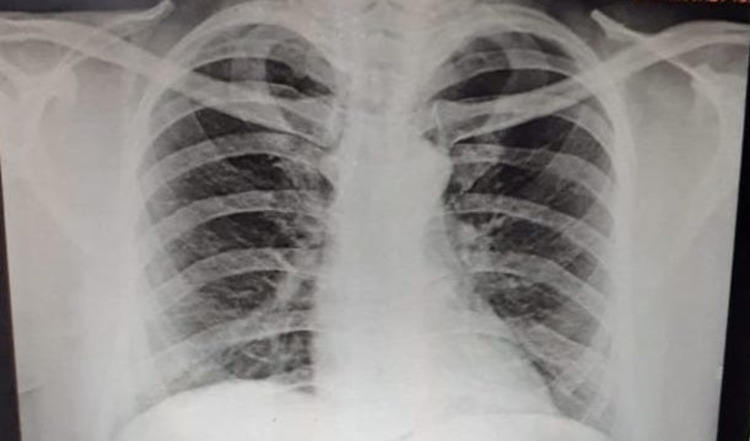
Chest X-ray showing diffuse patchy ground glass opacities in the bilateral lung fields.
